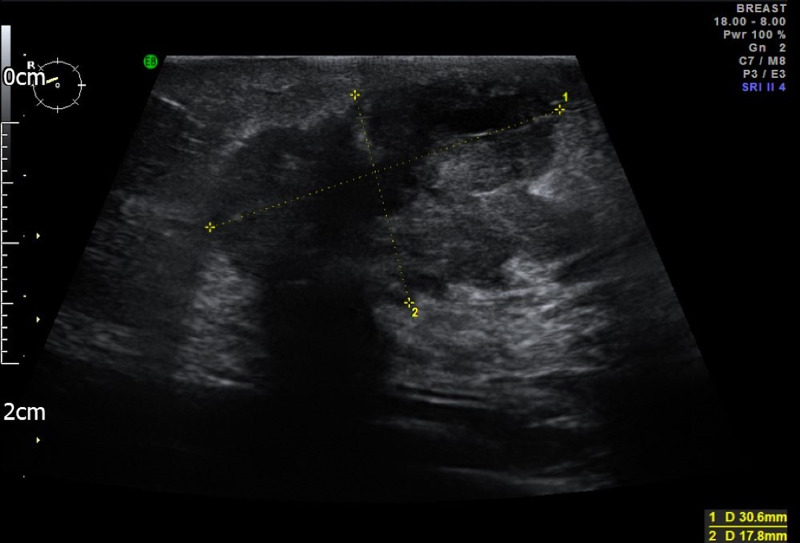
Ultrasound right breast reported a speculated diffuse hypoecoic lesion in the right breast lower outer quadrant with an appearance suggestive of a neoplastic lesion
